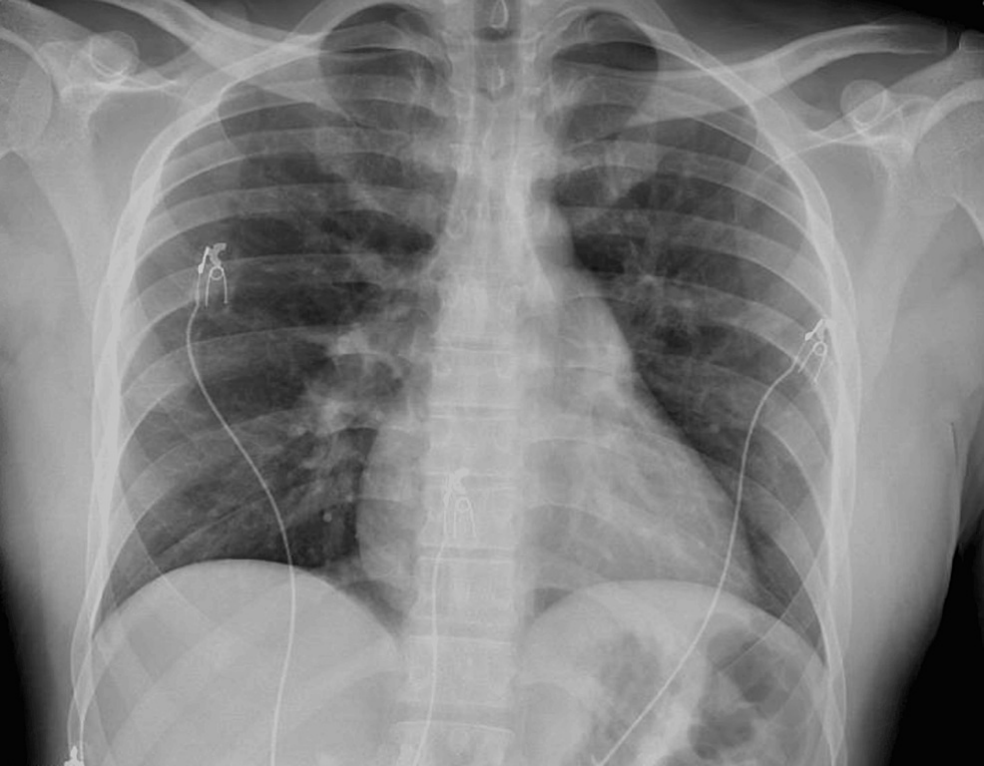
Chest X-ray showing no abnormalities
